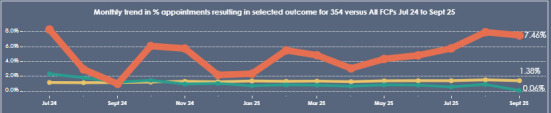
This graph shows MRI trends of all FCPs (green), FCPs from the same provider (yellow) and individual FCP (red) over the time we have data for this FCP. FCP, First Contact Physiotherapy.
